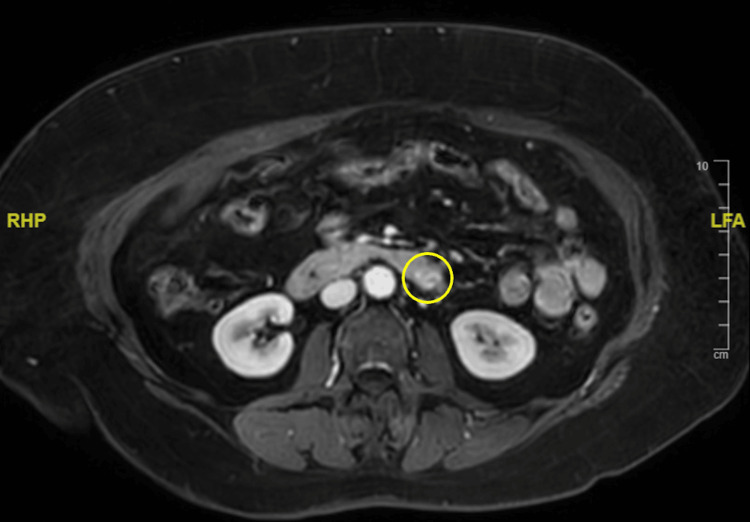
MRI scan (T2 weighted) of the abdomen Yellow circle: Outline of hypointense lesion of the pancreatic cauda
